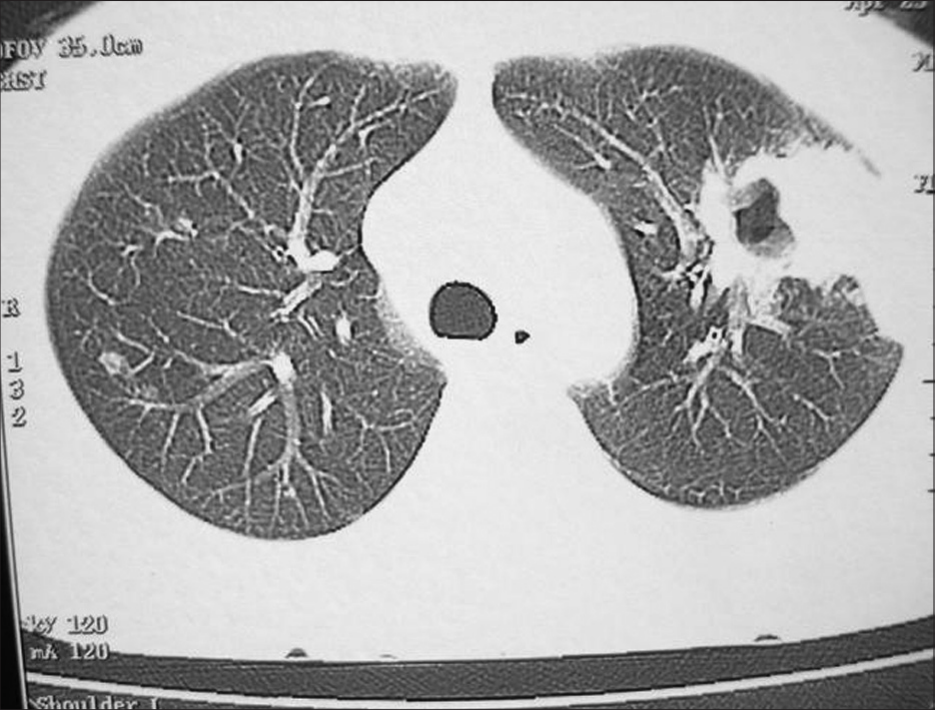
Computed tomography of thorax revealed fibro-consolidation with cavitation in anterior segment of left upper lobe with fibrotic nodule in anterior and posterior segment of right upper lobe
